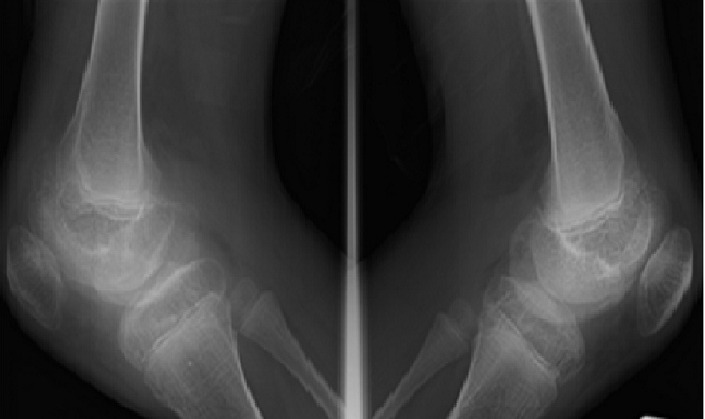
Enfant 08 ans, hémophile A sévère. Radiographie comparative des genoux en incidence de profil montrant une hypertrophie des noyaux épiphysaires condyliens fémoraux prédominant à droite
